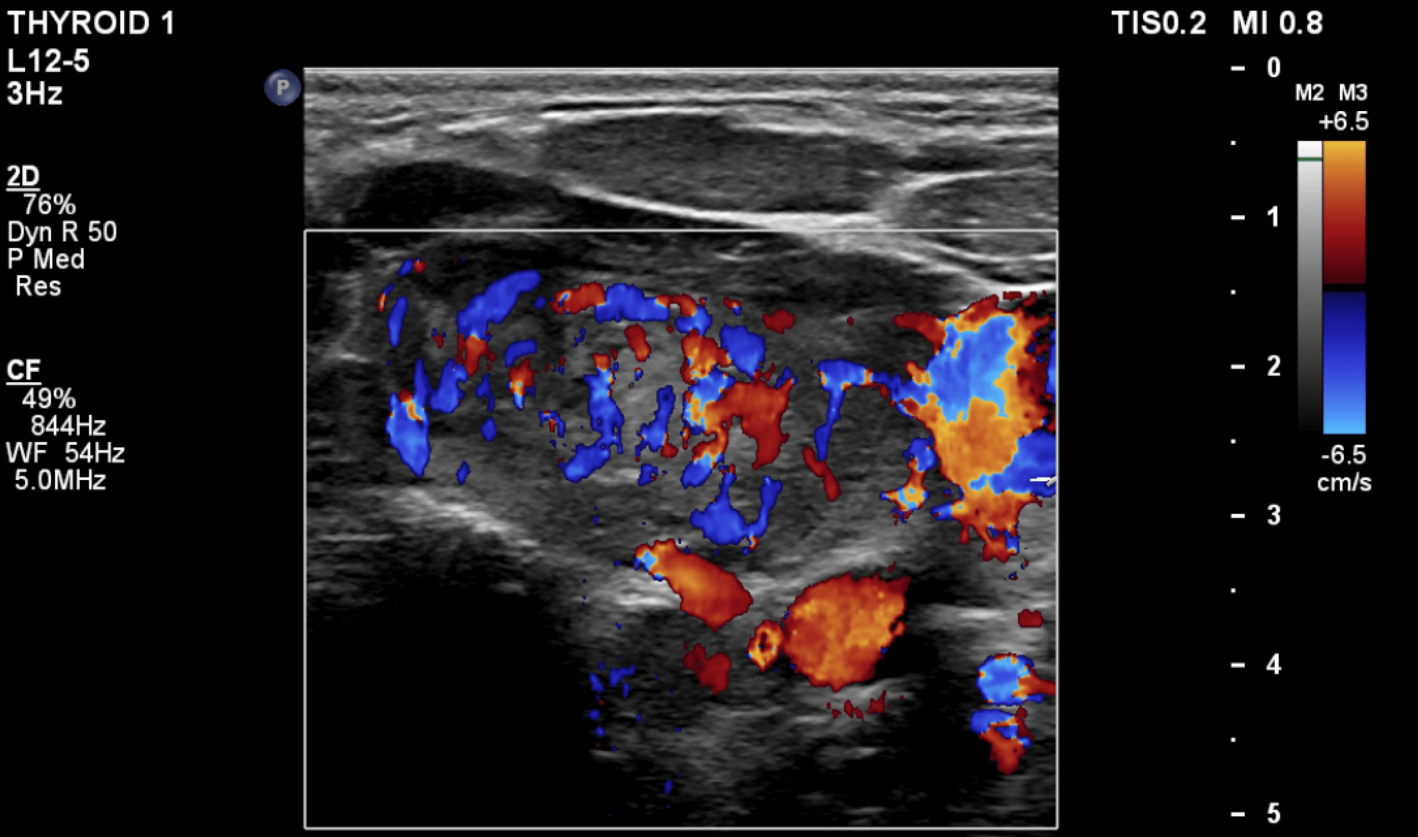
The patient's thyroid ultrasound with color Doppler shows increased blood flow consistent with thyroiditis.
